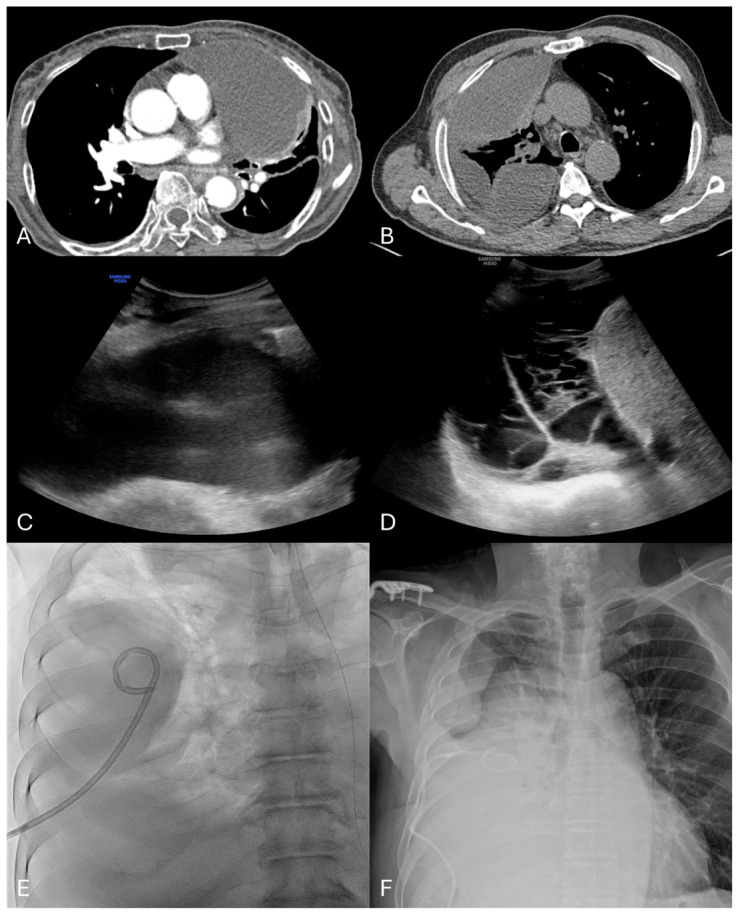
Representative imaging of study inclusion criteria and procedural guidance. (A) Computed tomography (CT) demonstrating a unilocular left-sided pleural effusion with a single well-defined locule. (B) CT demonstrating a multilocular right-sided pleural effusion. (C) Ultrasound (US) image showing an anechoic loculated pleural effusion. (D) Ultrasound image showing an echogenic pleural effusion with internal debris and septations. (E) Fluoroscopic image obtained during a US + Fluoroscopy-guided percutaneous catheter drainage procedure, confirming real-time pigtail catheter placement within the target pleural locule. (F) Post-procedure chest radiograph demonstrating satisfactory catheter position within the pleural space. All images are from representative study patients.
